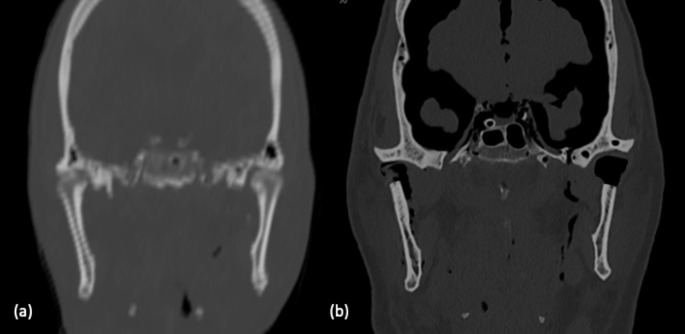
(a) Preoperative CT scan in frontal view; (b) postoperative CT scan in frontal view
